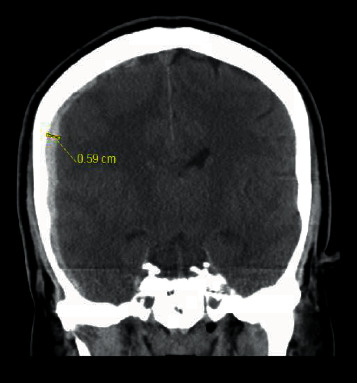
Noncontrast coronal CT head showing right crescent-shaped subdural blood collection measuring up to 6 mm in thickness.
